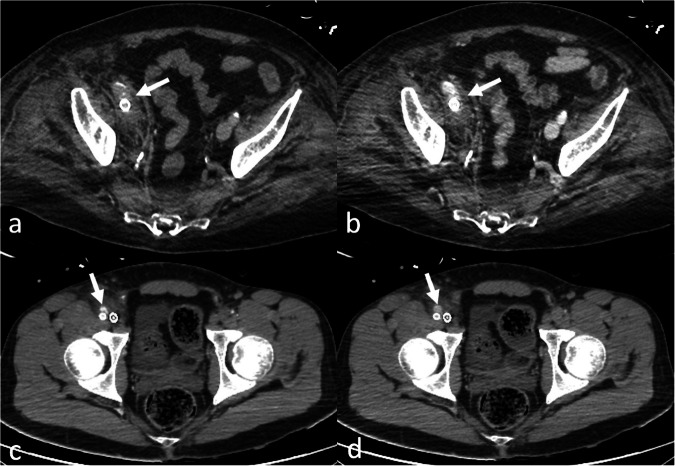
Axial computed tomography (CT) images unenhanced (a) and in the venous phase (b) reveal a blood collection adjacent to the right iliac vein (arrows), near the drainage cannula. In b, there are no signs of active contrast medium extravasation. Axial CT images in the arterial (c) and late (d) phases show a pseudoaneurysm of the right common femoral artery at the site of arterial return cannula access for veno-arterial (VA) extracorporeal membrane oxygenation (ECMO) (arrows), surrounded by a perivascular hematoma
